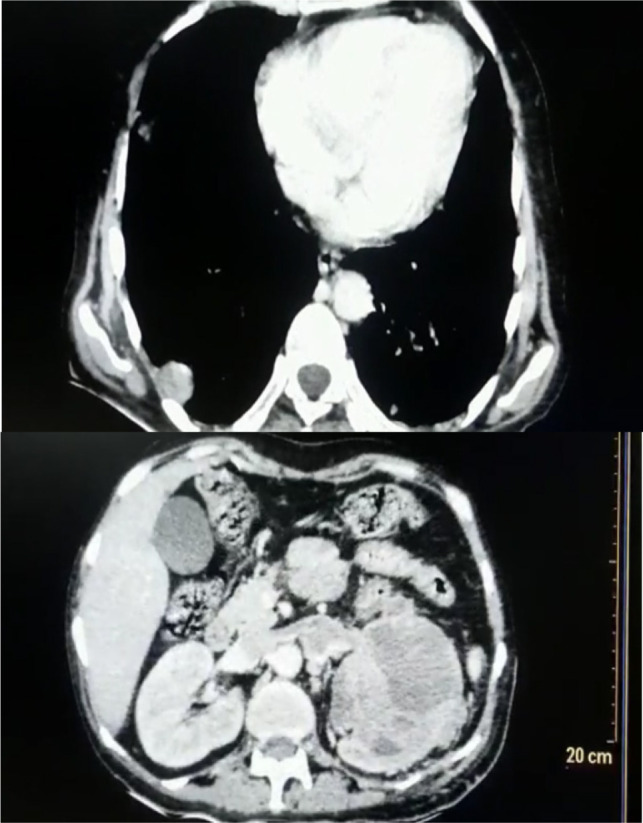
Computed tomography chest with abdomen.
